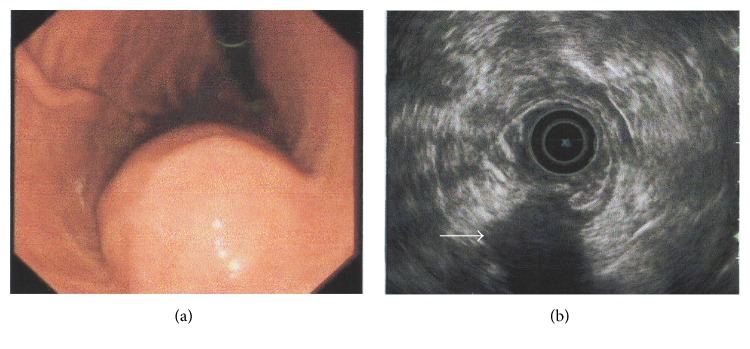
Endoscopic (a) and endosonographic (b) findings in the current case of gastric schwannoma. (a) A round, submucosal mass with an indistinct border was observed at the lesser curvature of the gastric body. (b) On endoscopic ultrasonography, the lesion (white arrow) appeared homogeneous and its echogenicity was lower than that of the normal muscle layer. The mass measured 3.7 × 3.2 cm and originated from the fourth layer.
